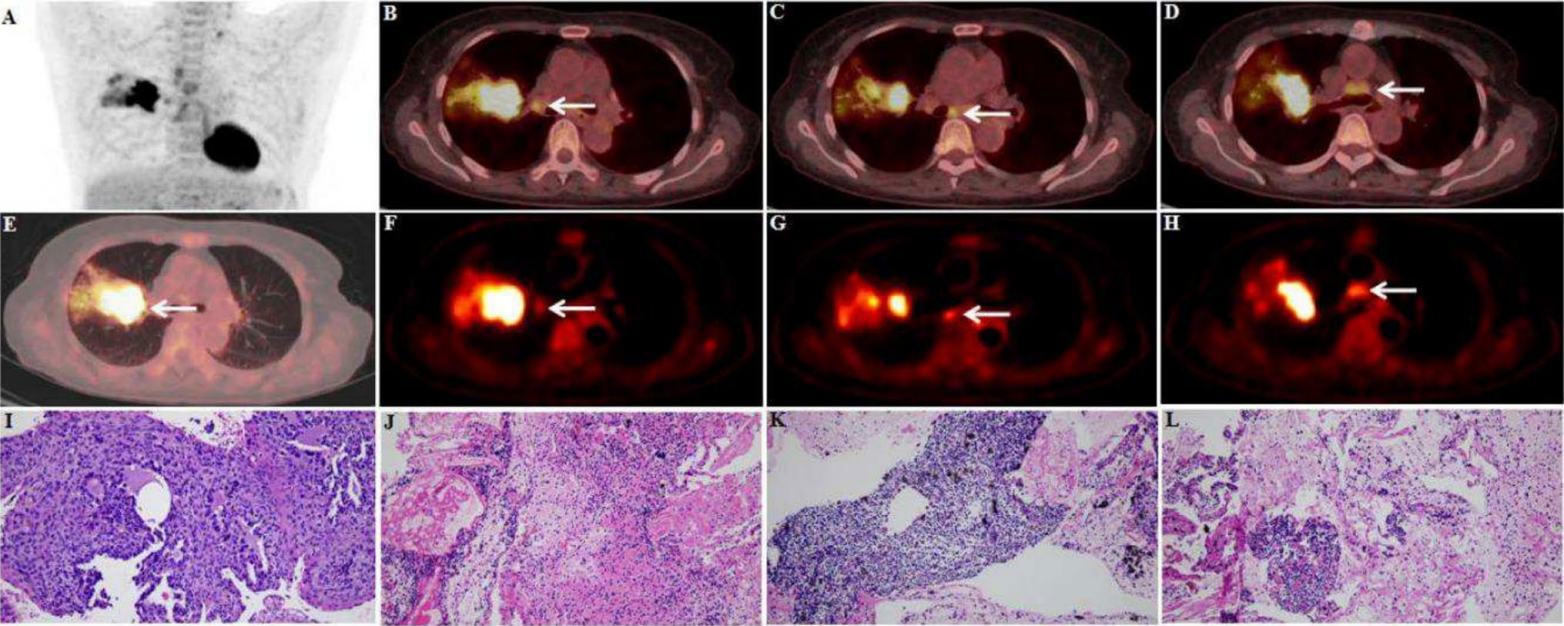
dPET/CT and sPET/CT images of non-metastatic FDG-avid LNs
